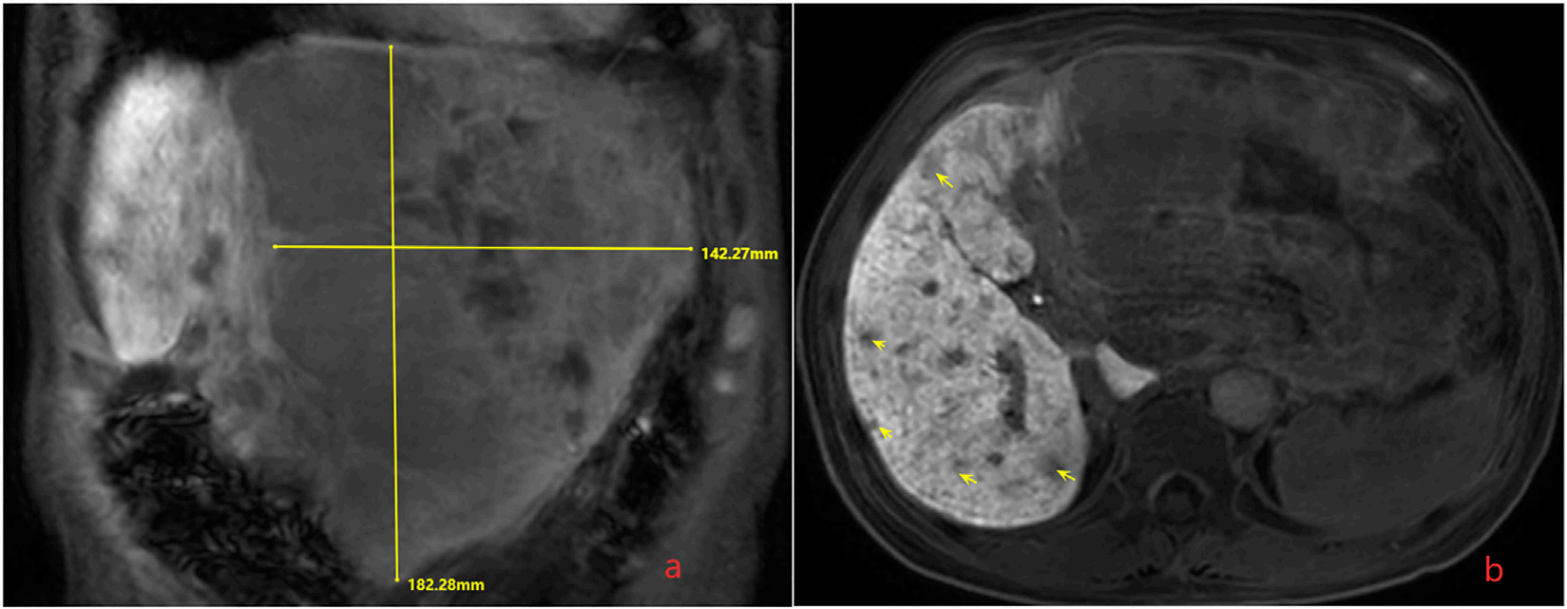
Magnetic resonance imaging (MRI) shows the tumor in the left lobe of the liver with multiple metastases in the liver, and with tumor thrombosis in the left portal vein. (A) The huge tumor in the left lobe of the liver; (B) the multiple metastases in the right lobe of the liver.
